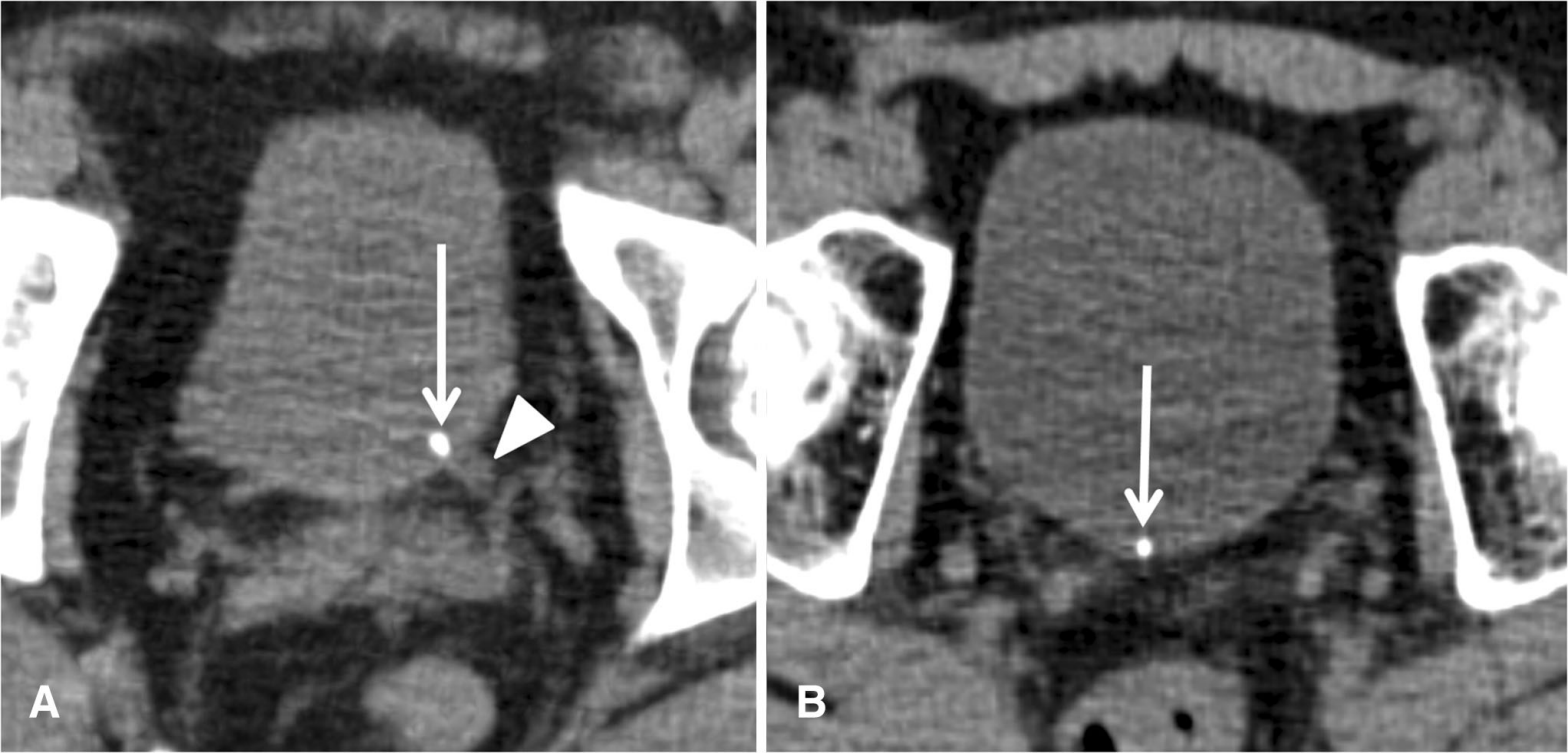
A, B Supine axial unenhanced CT images in two different patients with acute left-sided renal colic and a stone clearly impacted at the UVJ (*arrow* in A), dilated distal left ureter (*arrowhead* in A), and a ureteral stone that had unequivocally already passed into the urinary bladder (*arrow* in B) and is located posteriorly in the bladder more to the midline
